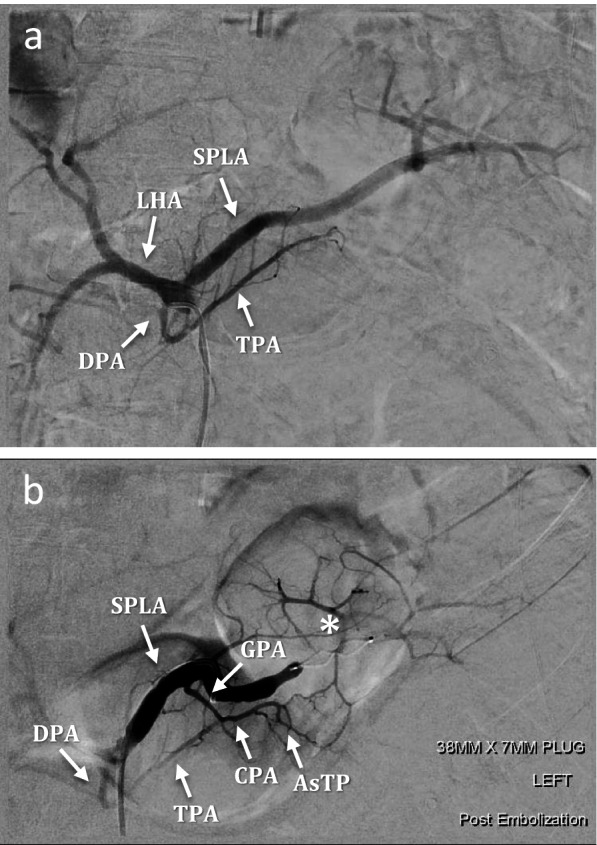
PSAE in patient presenting with blunt splenic trauma and variant splenic anatomy. a Pre-embolisation diagnostic angiogram revealed the DPA arising directly from the coeliac trunk along with the left hepatic artery (LHA). CT angiography showed the right hepatic artery origin from the superior mesenteric artery, and the left gastric artery arising from the coeliac trunk, not shown here. b Post-embolisation angiogram shows an AVP plug (white star) inserted distal to the GPA origin and splenic perfusion maintained via the AsTP collateral circulation. This case was thus considered a Type III embolisation
